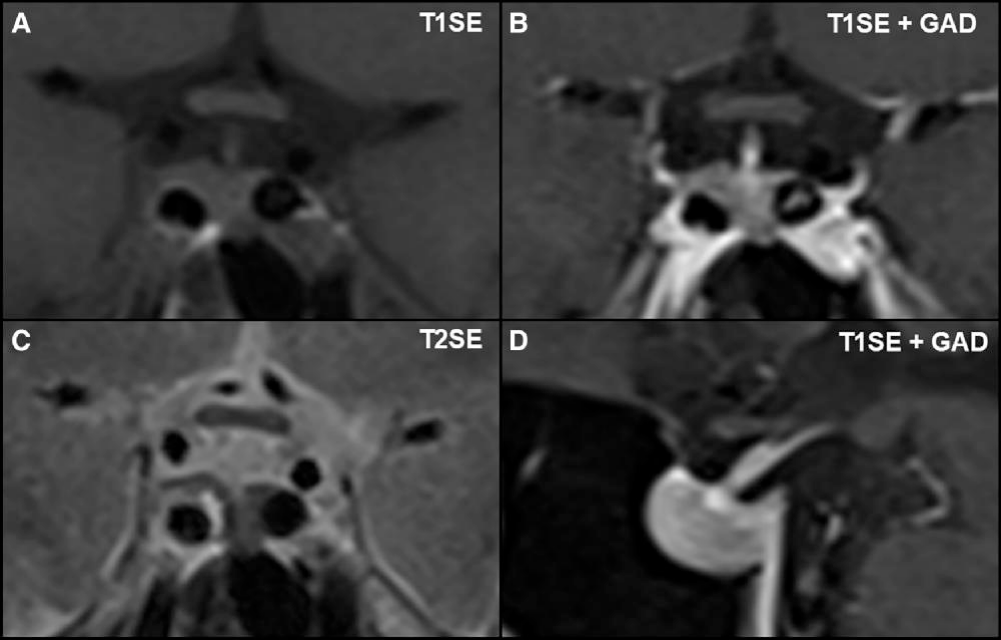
Pituitary MRI at initial presentation. No discrete adenoma is visible on T1-weighted coronal precontrast (A) and postcontrast (B), T2-weighted coronal (C), and T1-weighted sagittal postcontrast (D) sequences. The sellar anatomy appears asymmetric, consistent with a medially positioned left internal carotid artery.
